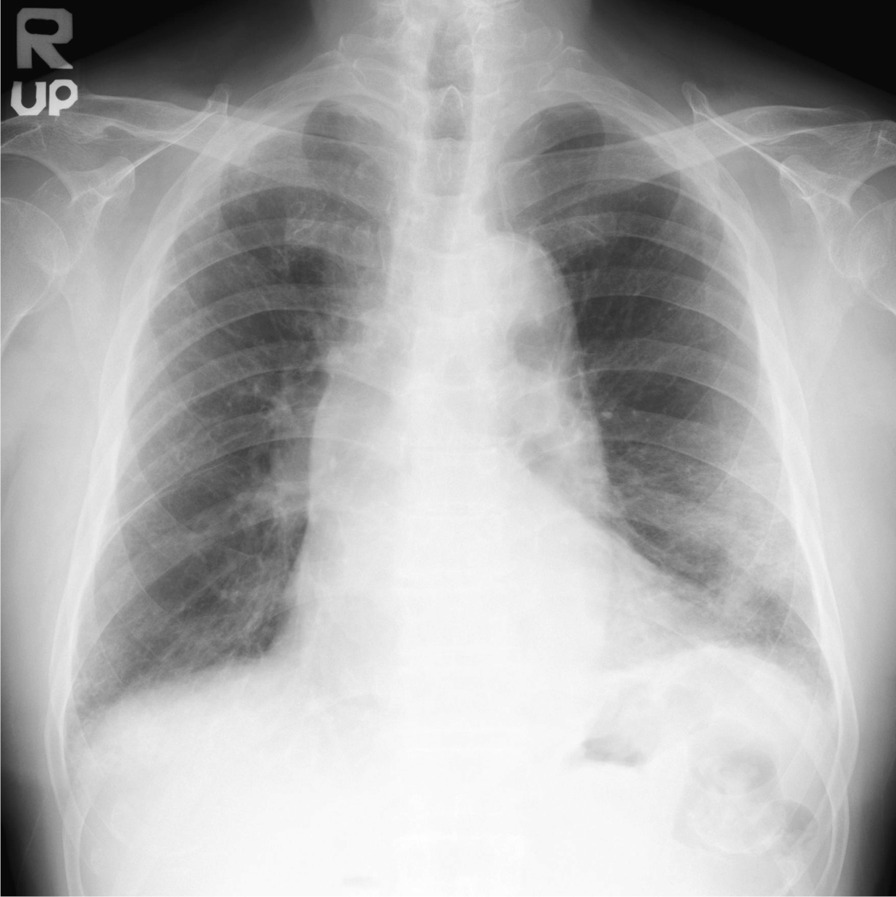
Chest X-ray at the first visit
